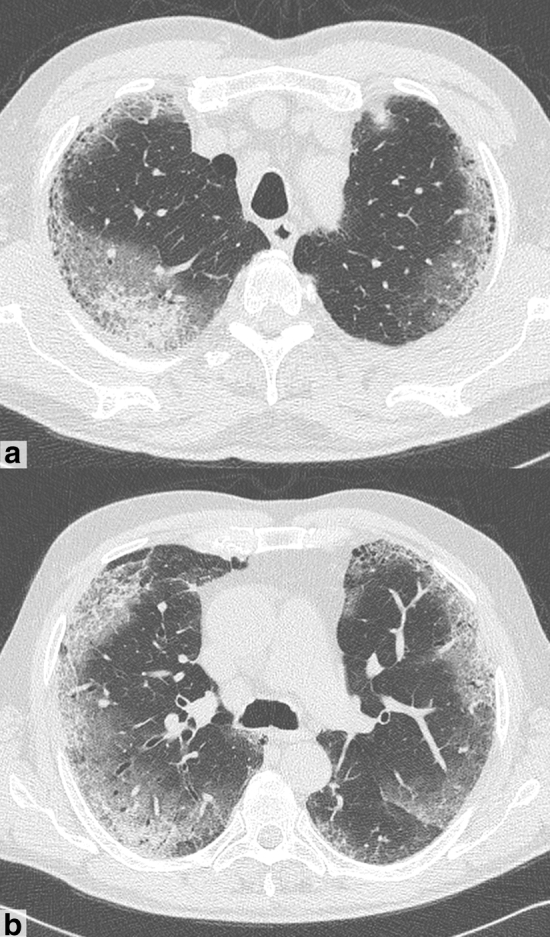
A 75-year-old man presented with fever and dyspnea in the last 13 days, COVID+. a–b CT shows diffuse bilateral ground-glass opacities with prevalent peripheral distribution, septal thickening and small areas of consolidation with air bronchogram (mixed GGO and consolidation pattern)
